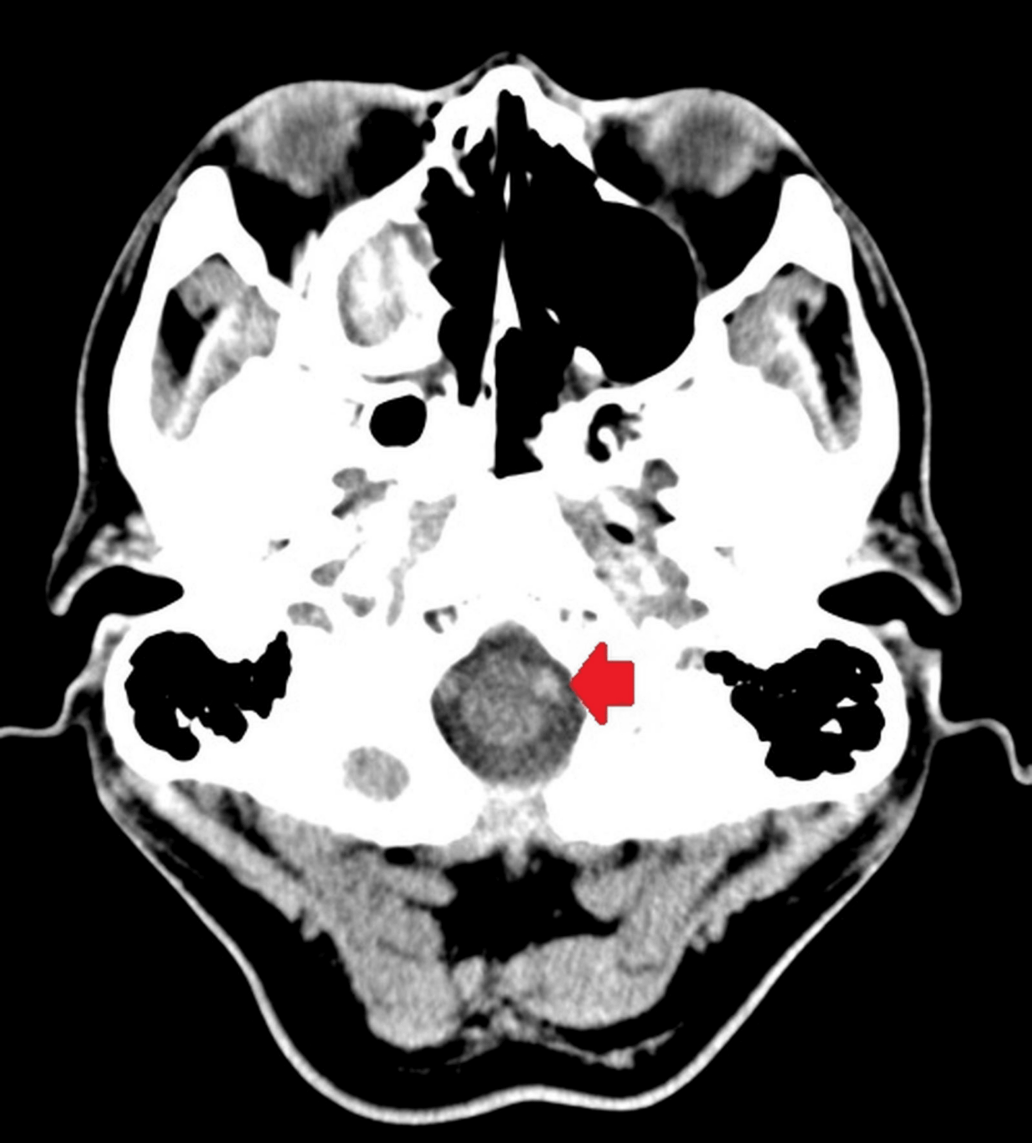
A non-contrast CT scan shows a hyperdense appearance of the left vertebral artery, indicating a hyperacute cerebral infarct image in the vertebral artery region (red arrow), suggestive of thrombus or dissection and hyperacute cerebral infarction.
